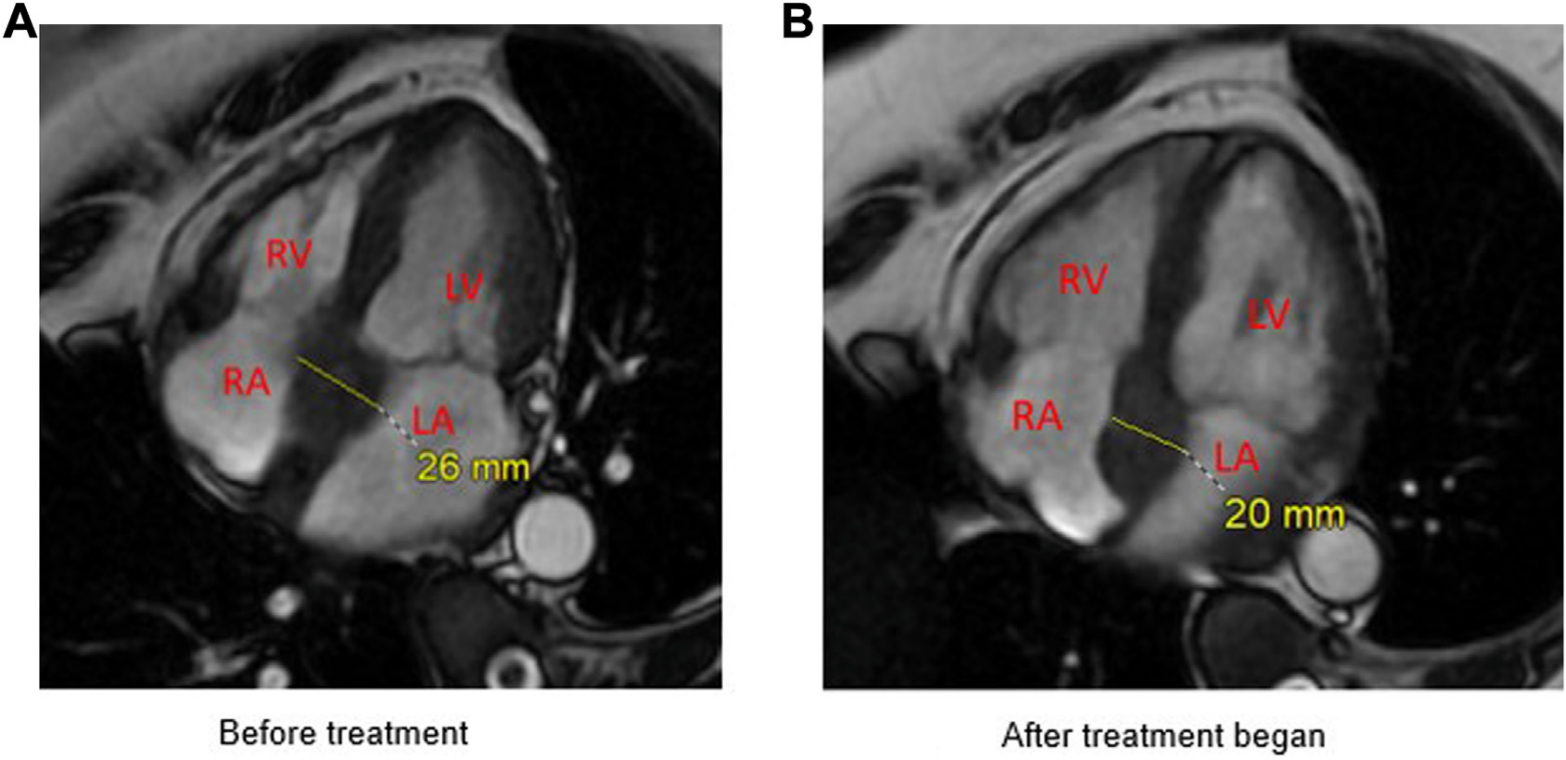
Cardiac magnetic resonance imaging; yellow lines denoting the size of the abnormal mass involving the interatrial septum. A: Before treatment. B: After treatment began. LA = left atrium; LV = left ventricle; RA = right atrium; RV = right ventricle.
